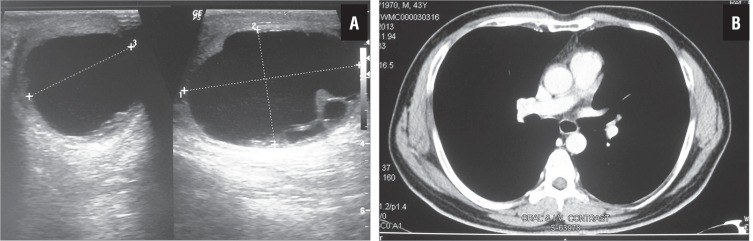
Scrotal ultrasound showing a cystic lesion with nodular soft tissue shadows at the periphery (a). CT abdomen one year post-surgery shows no lymphadenopathy (b).
